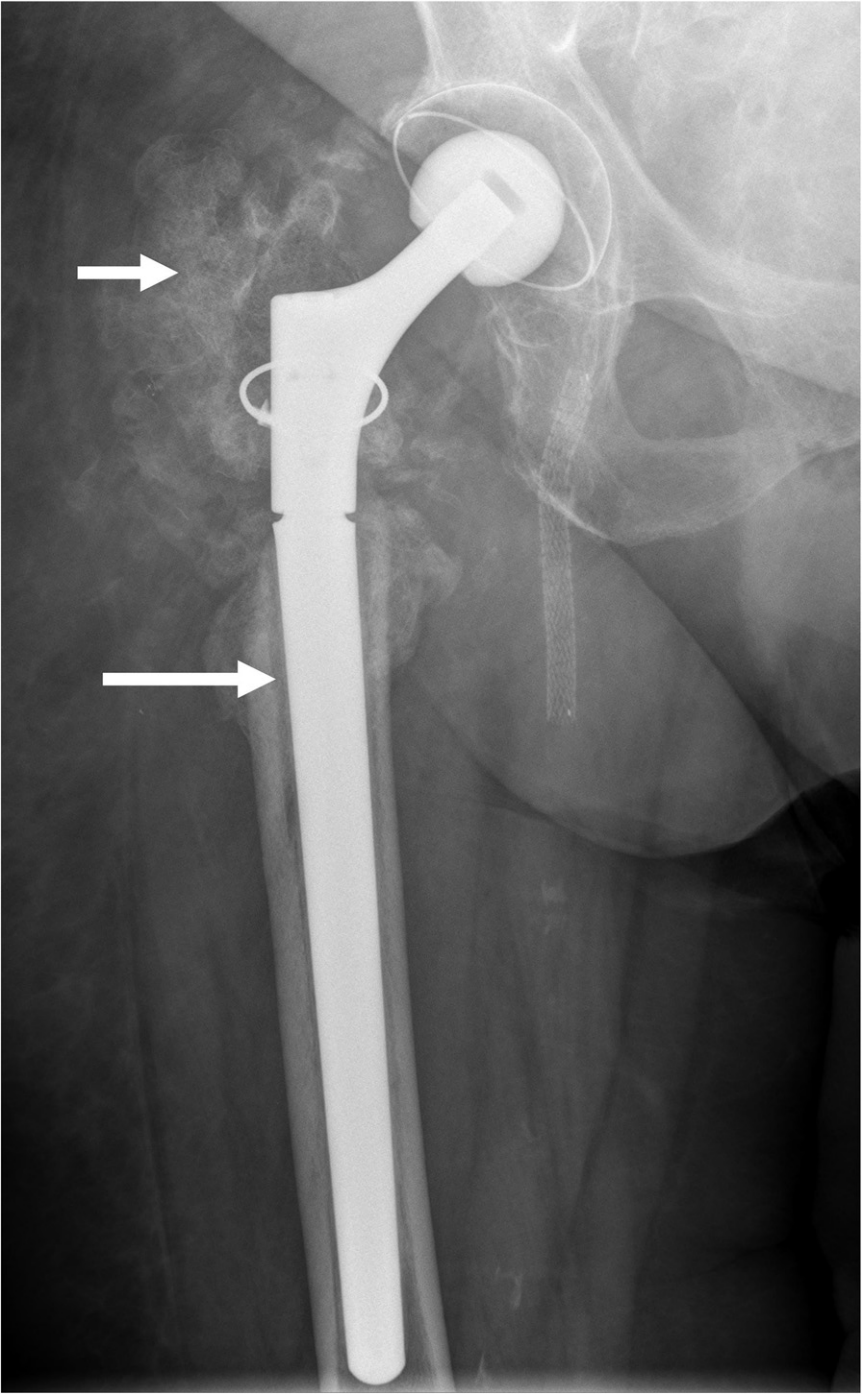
Conventional hip X-rays. Showing a conventional hip X-rays with existing infection with loosening of the implant (long bolt) and periarticular ossifications (short bolt)
